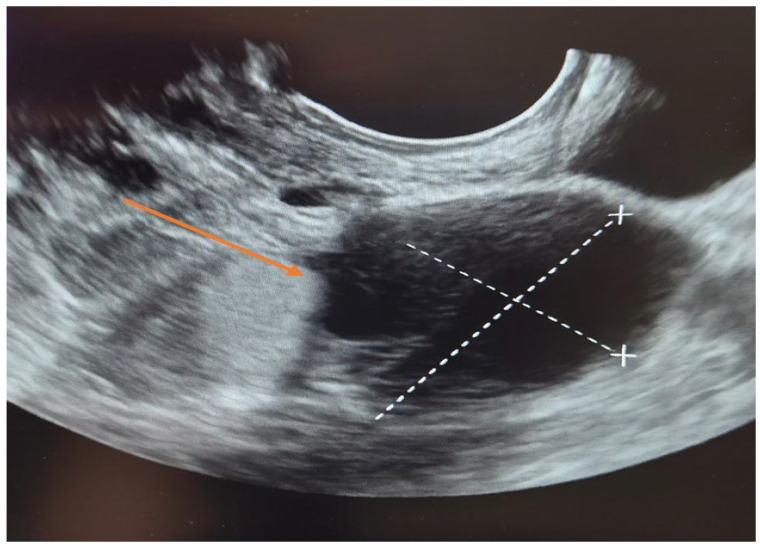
Transvaginal sonography (TVS) of the left adnexa ( orange arrow) demonstrating a complex ovarian cyst with cystic morphology, thin internal septations, and well-defined borders.
